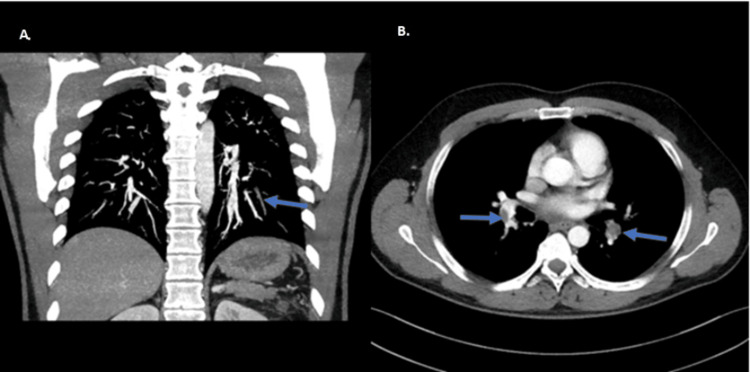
Computed tomography pulmonary angiography revealing unilateral subsegmental pulmonary embolism (blue arrows). A: coronal plane and B: axial plane
